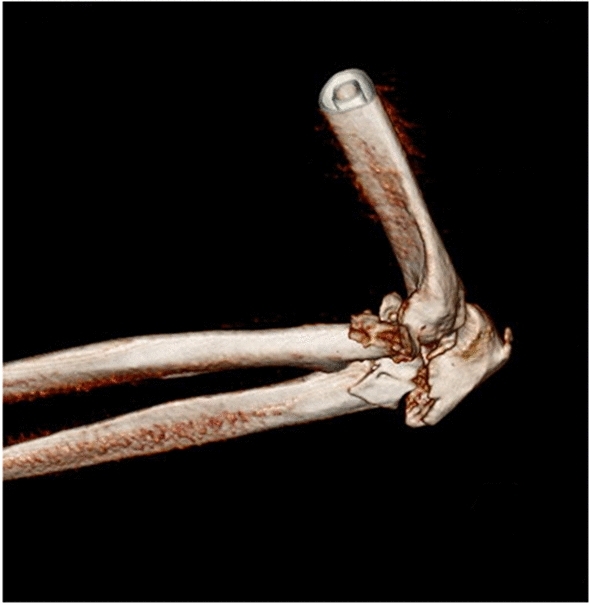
CT three-dimensional reconstructed sagittal image of a right sided comminuted proximal ulna and radial head fracture
